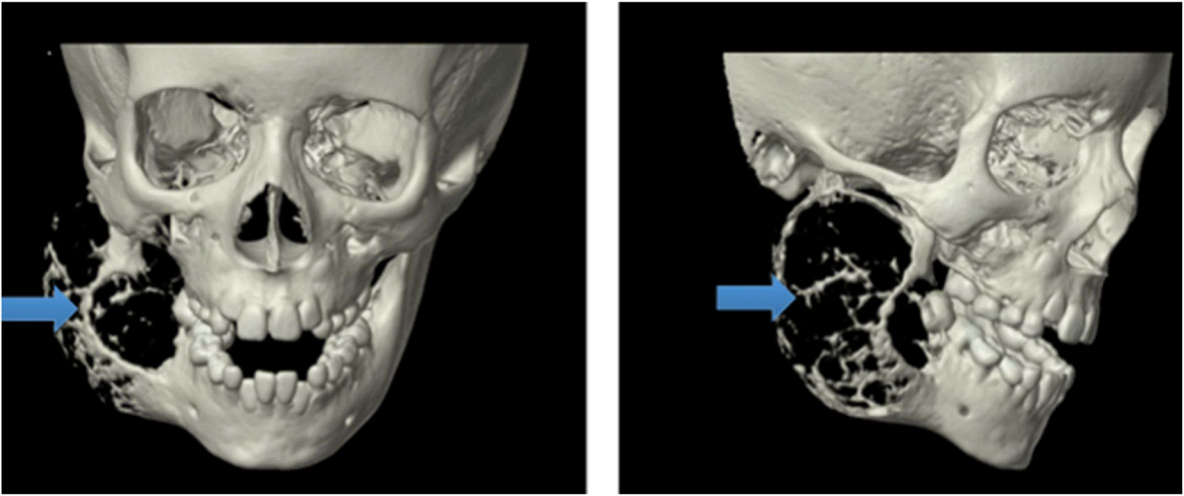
3D CT reconstruction of skull at second presentation to the emergency department. Arrows are pointing to lesion. Significant obliteration of the right hemimandbile including condyle
